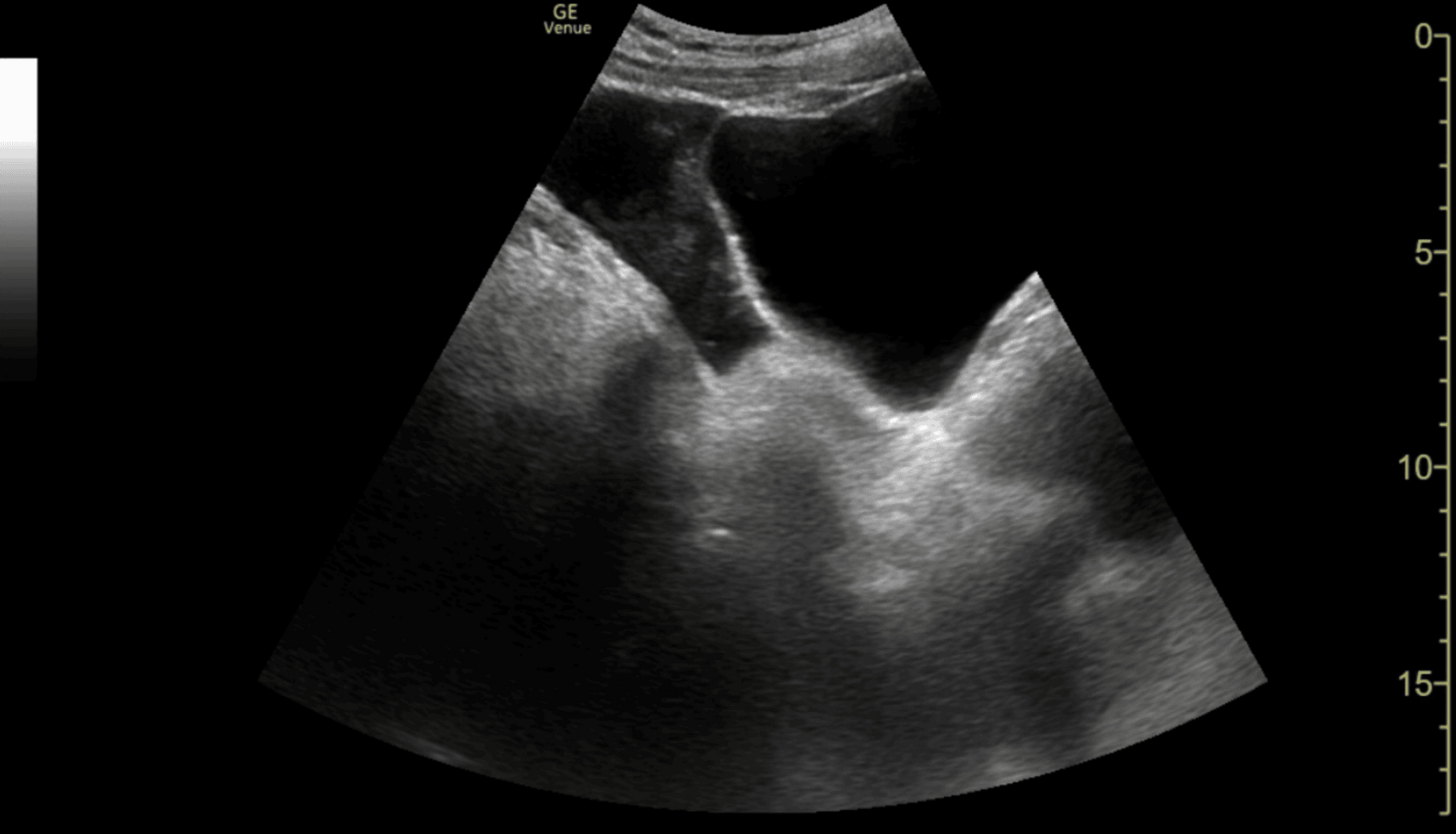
FAST scan, suprapubic longitudinal view, demonstrating free fluid collecting posterior to the bladder FAST: focused abdominal sonography in trauma
